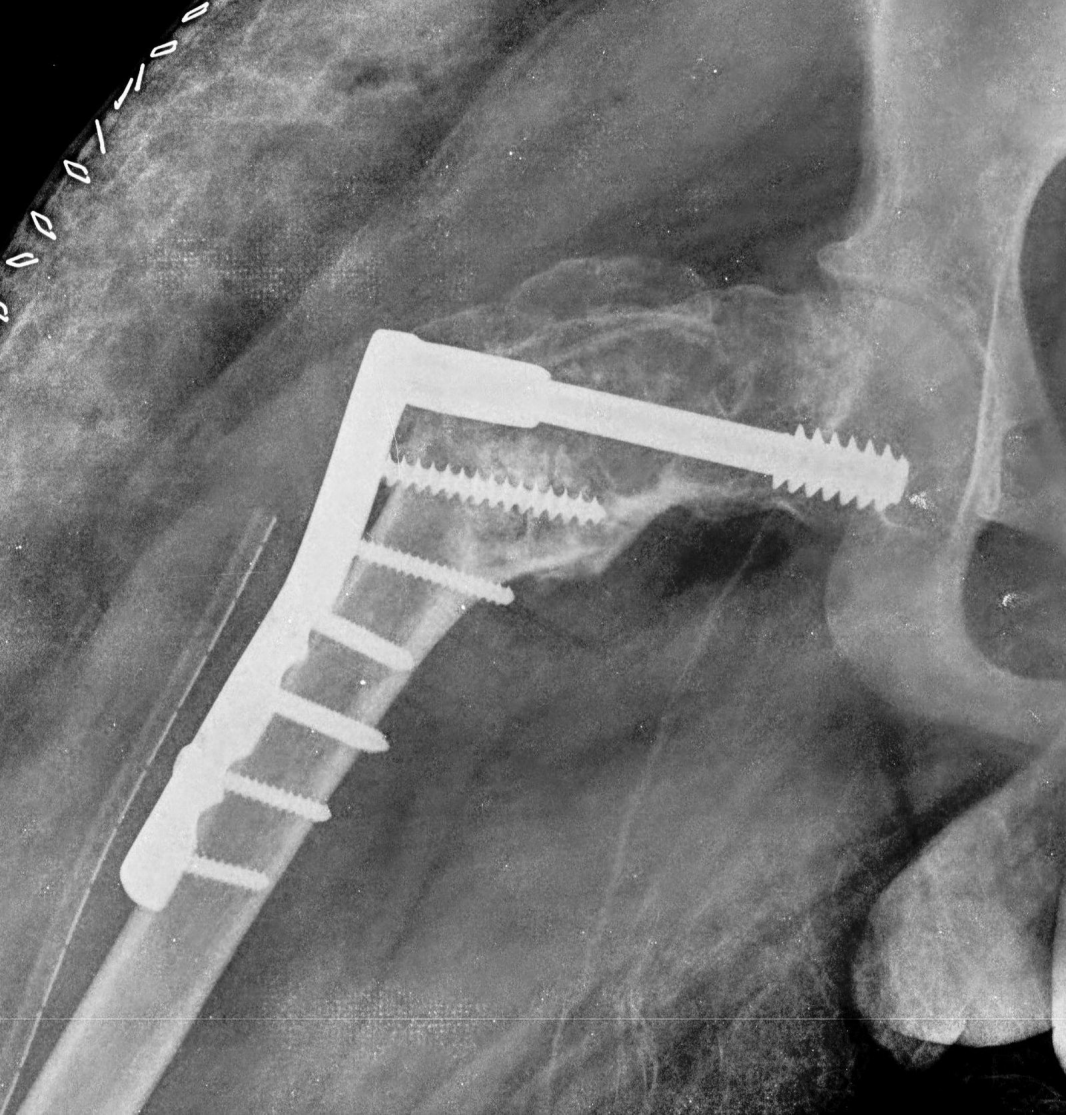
Anteroposterior X-rays of the hip with thigh, immediately post op showing a valgus osteotomy at the subtrochanteric region and fixed with a 95 degree Dynamic condylar screw (DCS) and barrel plate
